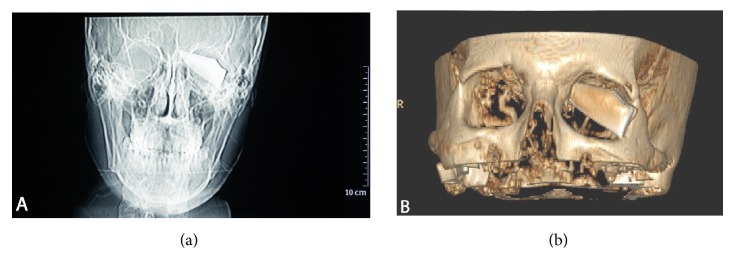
(a) Cranial X-ray showed a fragment of a dagger located intraorbitally in the superior and nasal quadrant and intracranially in the ethmoidal sinus in left site (including ruler for measurement). (b) The CT scans showed an intraorbital and intracranial foreign body of 4.6 cm x 2 cm in the left site.
